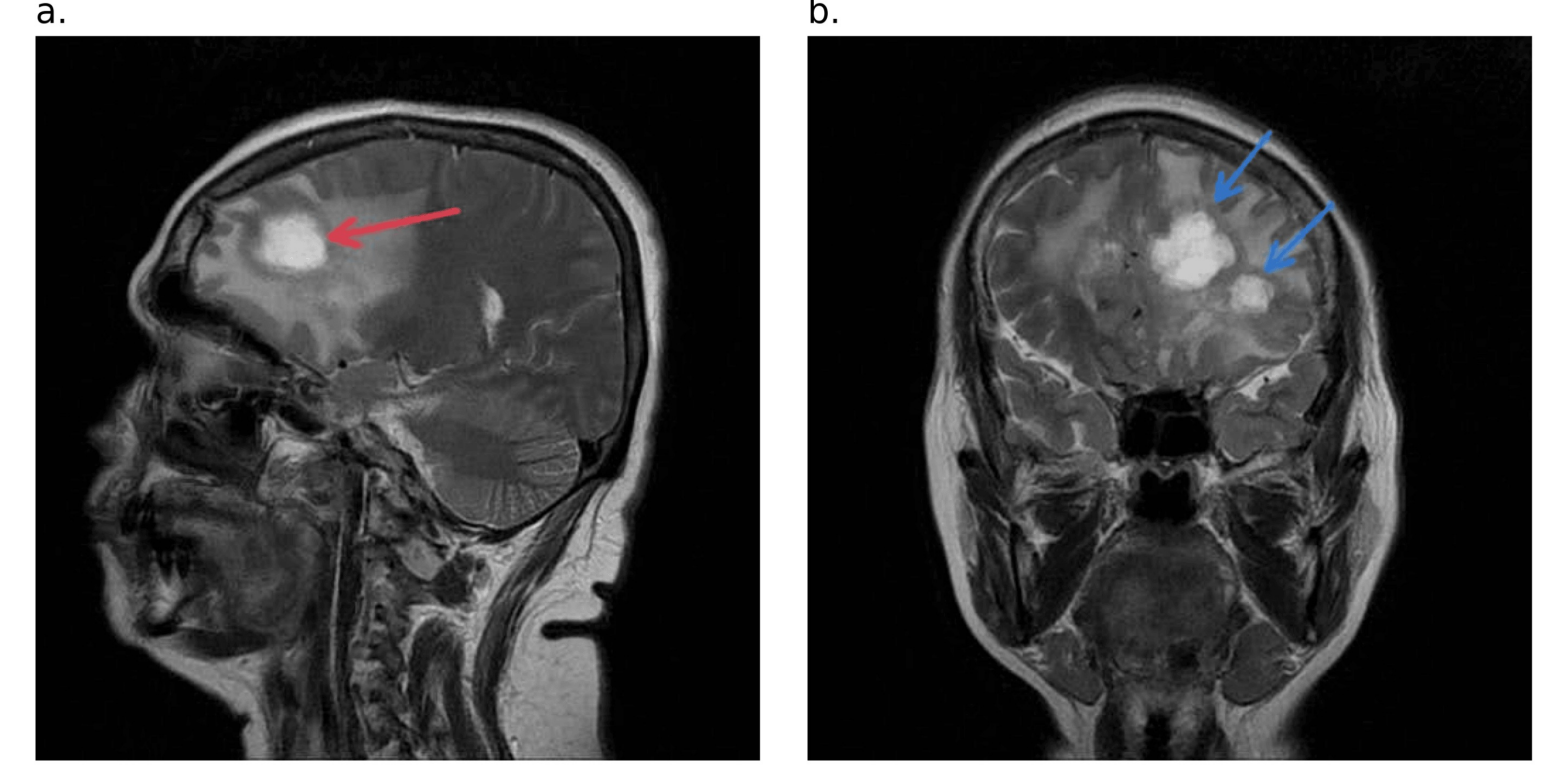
T2-weighted MRI image a. Sagittal view showing tumor (red arrow) in the anterior lobe. b. Coronal view showing multiple tumors (blue arrows) within the left anterior frontal lobe MRI: magnetic resonance imaging
